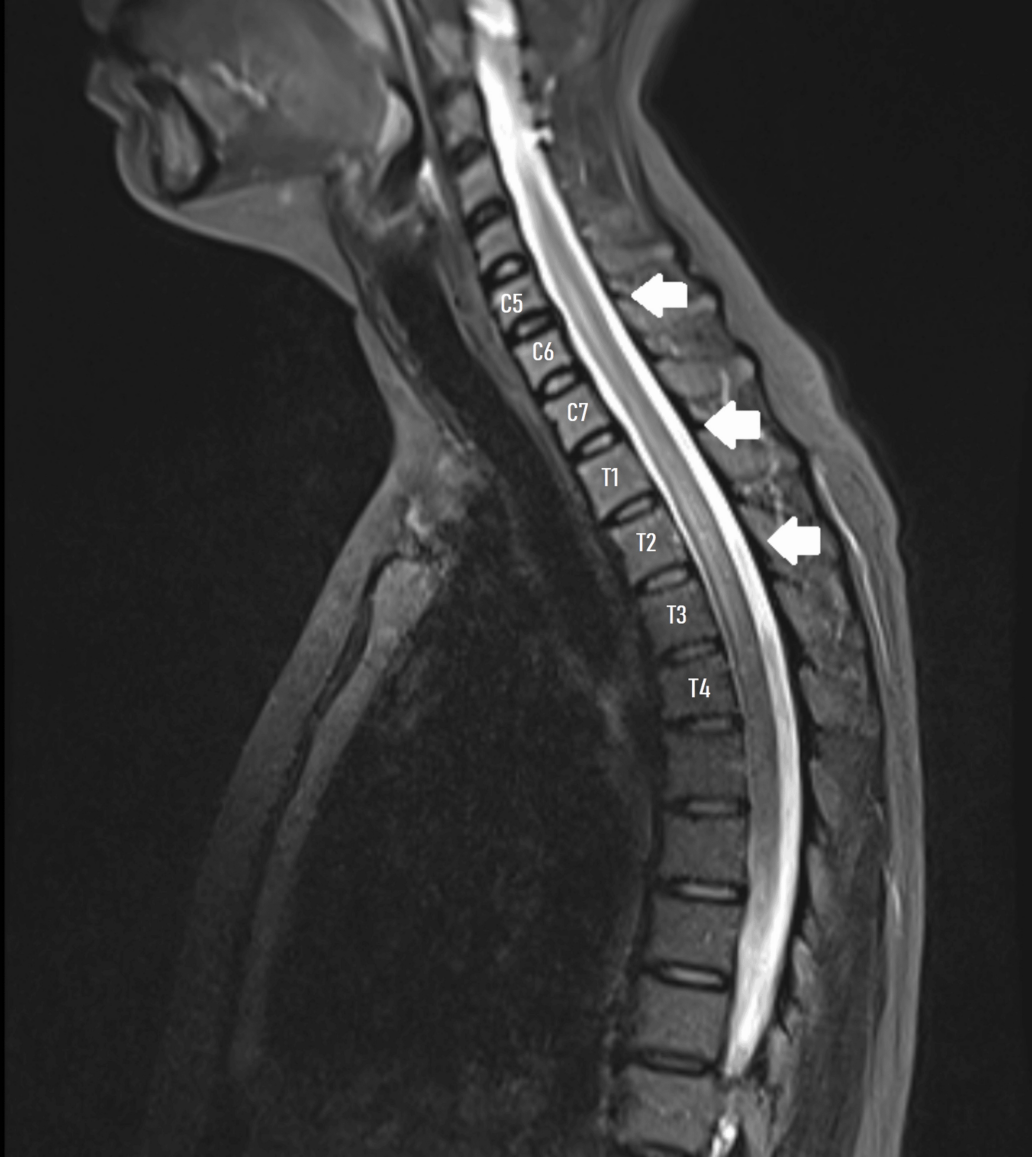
Longitudinally Extensive Spinal Cord Lesion Sagittal T2-weighted MRI of the cervical and upper thoracic spine showing a centrally located hyperintense lesion extending from C5 to T4, consistent with LETM (White Arrows), No gadolinium enhancement was seen (not shown).
